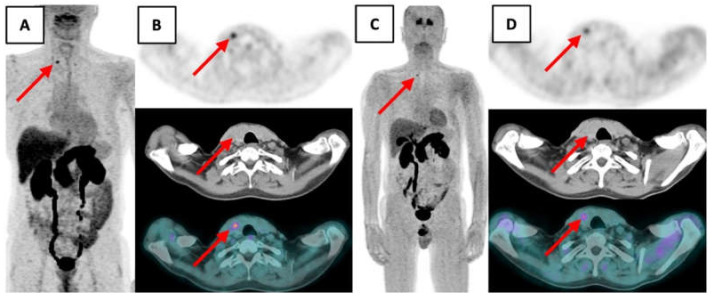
^18^F-FDOPA PET/CT showed a punctiform focus of the right thyroid lobe at the superior part of the nodular bulge suspected of MTC (red arrow).(A) Maximum intensity projection 12 min post-injection. (B) Transaxial PET, CT and fusion PET/CT 12 min post injection. (C) Maximum intensity projection 79 min post-injection. (D) Transaxial PET, CT and fusion PET/CT 79 min post injection.
